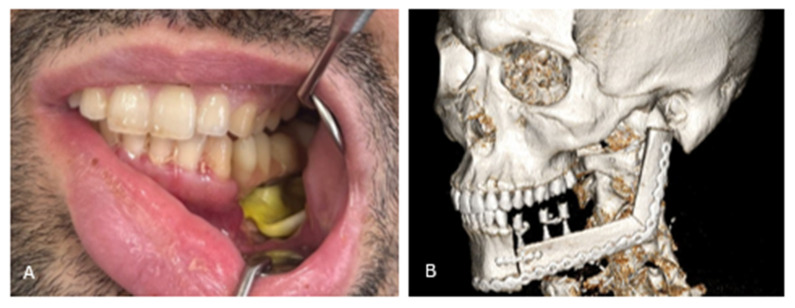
Clinical and radiological situation one week postoperatively. (A) Clinical picture with provisional screw-retained bridge. There was no dental occlusion posteriorly, most likely caused due to postoperative swelling. The Elemental^®^ wound dressing is visible under the provisional bridge and keeps the skin graft in place. (B) Aside from a small opening between the native mandible and the fibula graft, the postoperative CT scan shows a good alignment of the fibular graft with the native mandible. There is a diastasis between the distal end of the FFF and the glenoid fossa.
